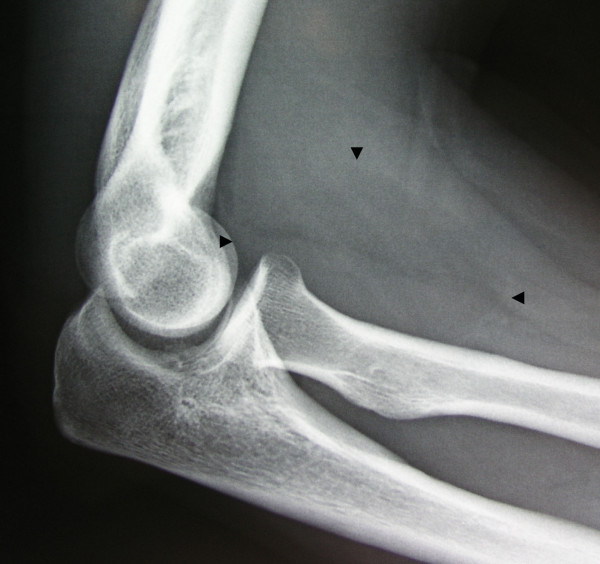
A lateral elbow X-ray subtly demonstrates a well circumscribed mass in the anterior proximal forearm (arrows).
